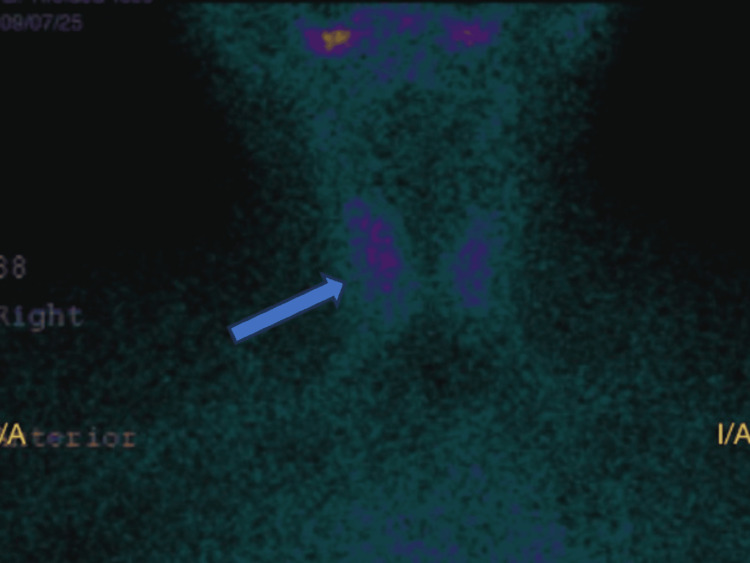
Thyroid scintigraphy demonstrating diffusely decreased uptake with focal cold areas in the inferior poles of both thyroid lobes (arrow), raising suspicion for possible occult thyroid pathology
